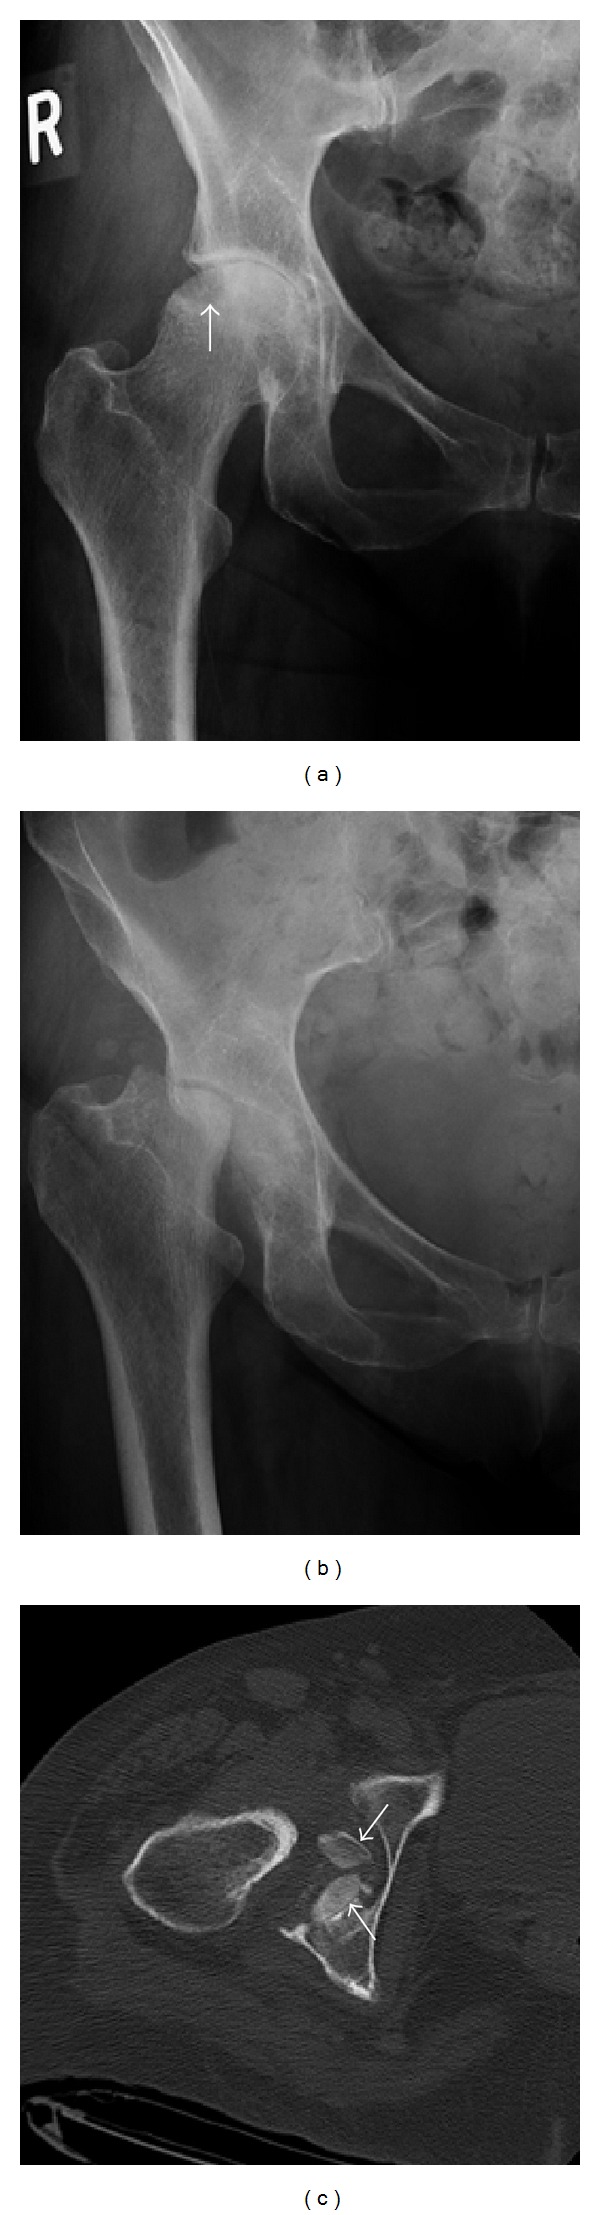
(a) AP radiograph of the right hip. A focal area of subchondral lucency is present involving the superolateral aspect of the right femoral head (arrow). (b) The follow-up radiograph taken 5 months later reveals near complete destruction of the femoral head. (c) CT scan of the right hip in the axial plane shows loss of the femoral head with two bone fragments within the hip joint (arrows).
